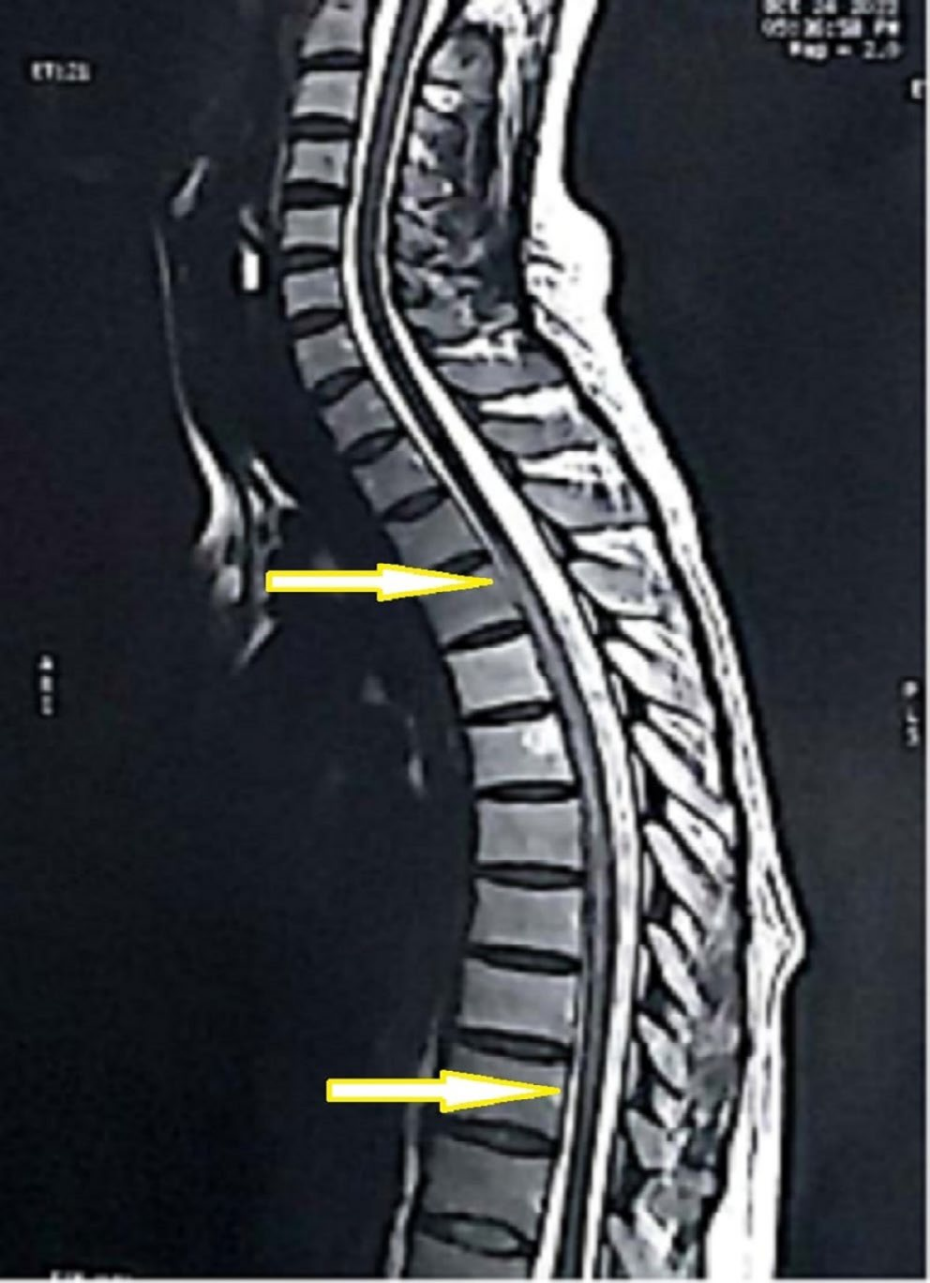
The patient's magnetic resonance imaging, sagittal view of the thoracic vertebrae; the arrows indicate the levels of syringomyelia between the fourth and tenth thoracic vertebrae.
